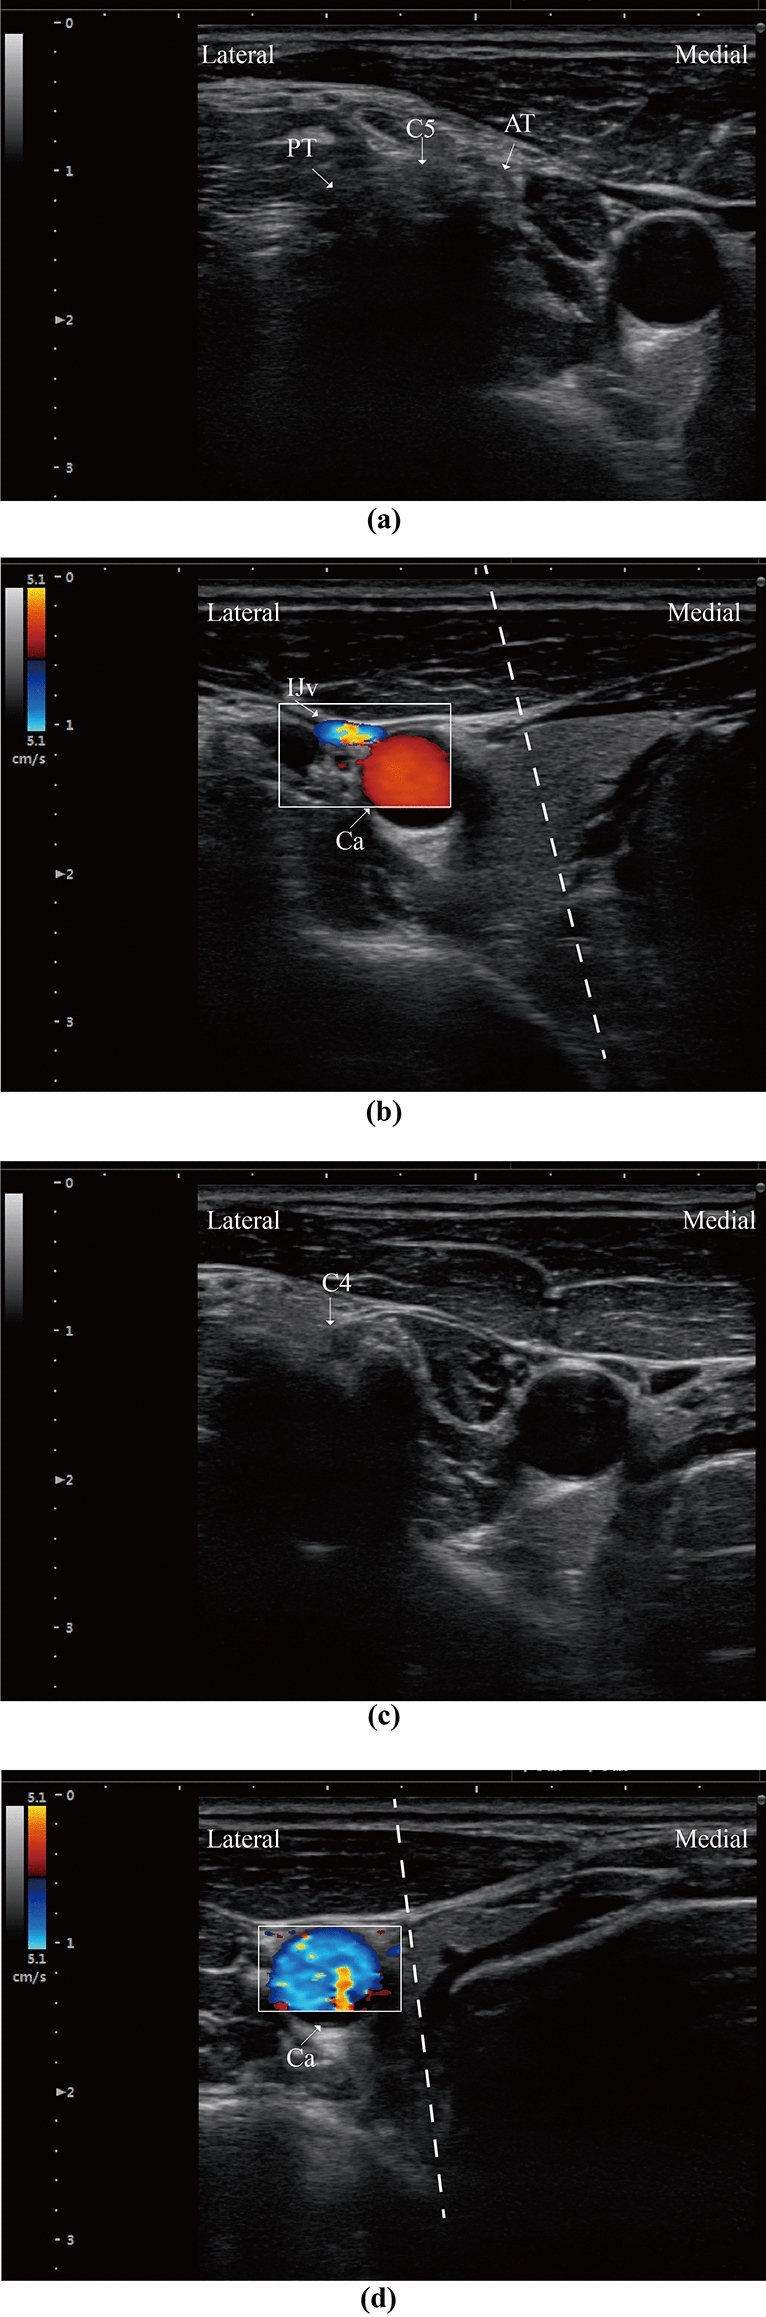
Ultrasound-guided cannulation for upper cervical intervertebral space. (a,b) Imaging scan of C5 level, and placement of cannula into the C4/5 disc. (c) Sonography of C4 cervical nerve root. (d) Cannulation pathway for C3/4 intervertebral disc.
